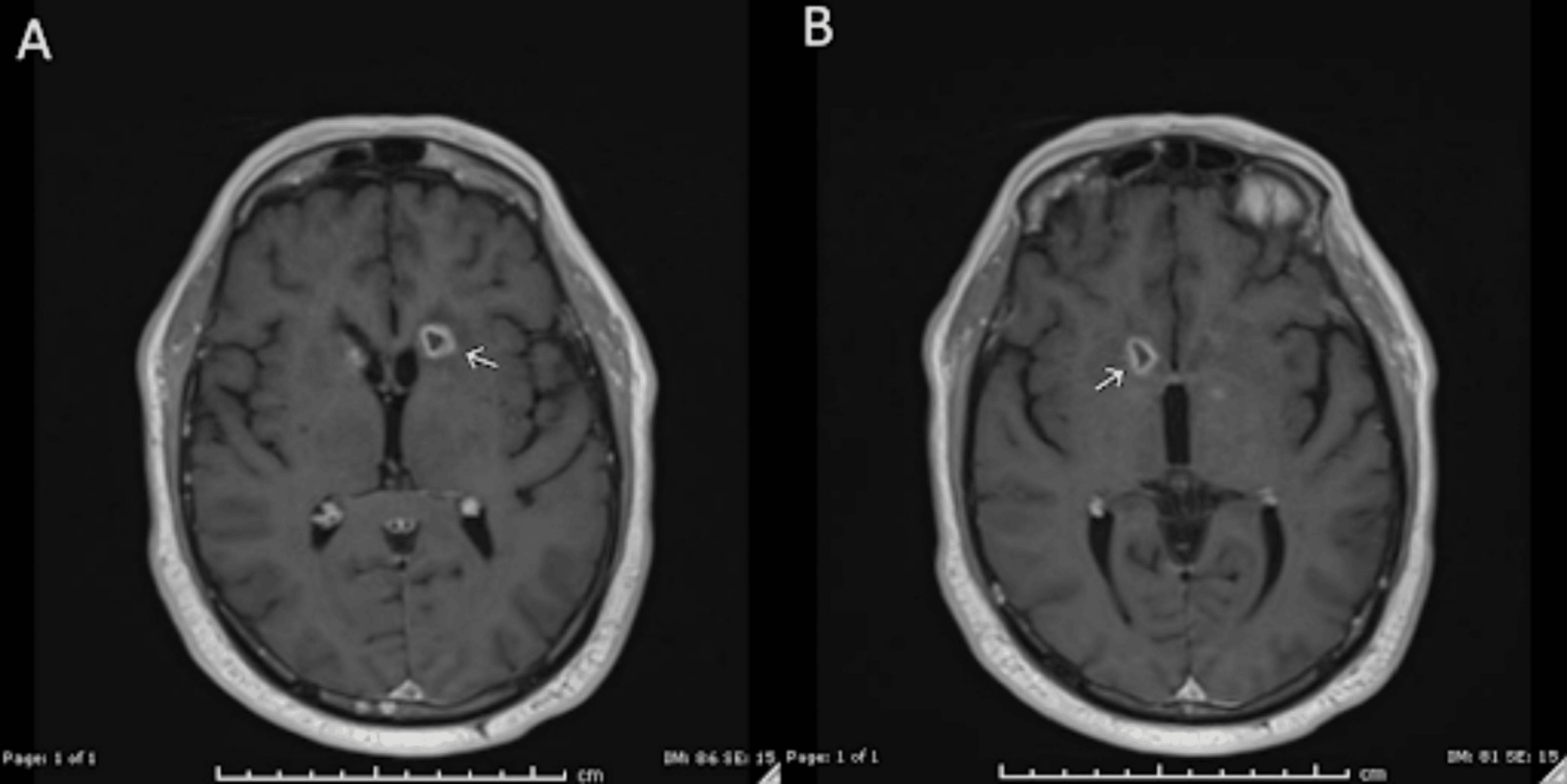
MRI T1 with contrast enhancement 99 days after the original MRI (A-B) Interval development of peripherally enhancing lesions in the bilateral caudate heads. MRI: magnetic resonance imaging
